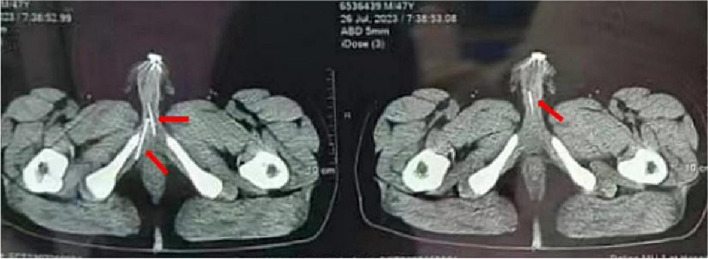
July 26, 2023, plain CT of the pelvis. The arrows show needles in the cavernous body of the penis on both sides
